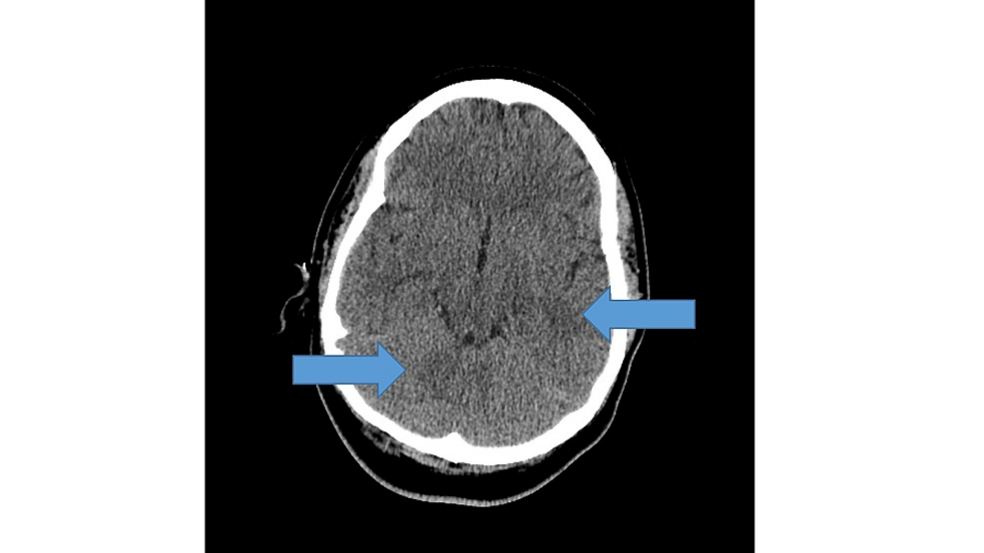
Computed tomography of the head showing subtle patchy hypodensities (arrows) seen in the basal ganglia as well as the parietal, occipital, and frontal lobes.
